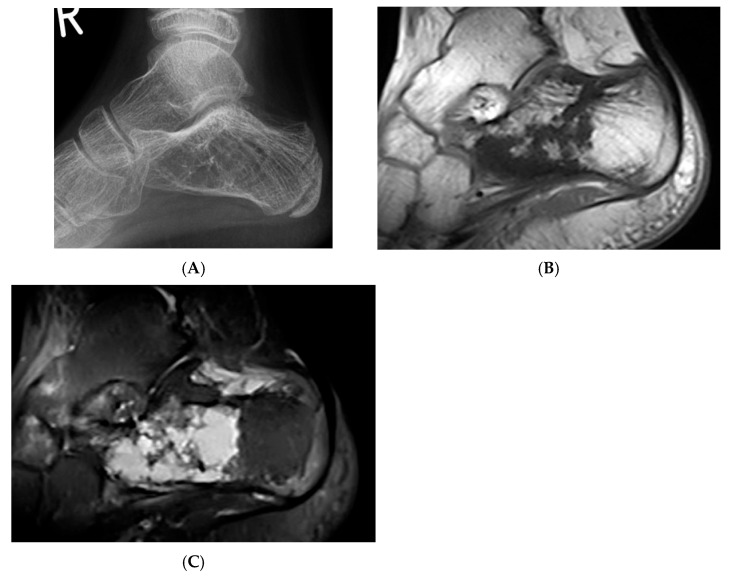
Haemangioma, calcaneum. (A) Plain radiograph demonstrating honeycomb pattern. (B) Sagittal T1W MRI and (C) sagittal STIR MRI demonstrating intralesional fat component (low T1 and high STIR) and septations.
